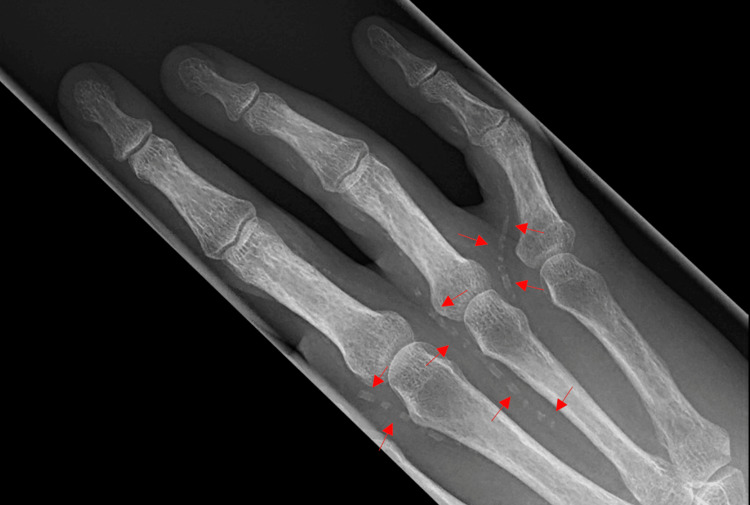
Calcification of peripheral vessels is evident on the hand X-ray superior view (shown by arrows).
